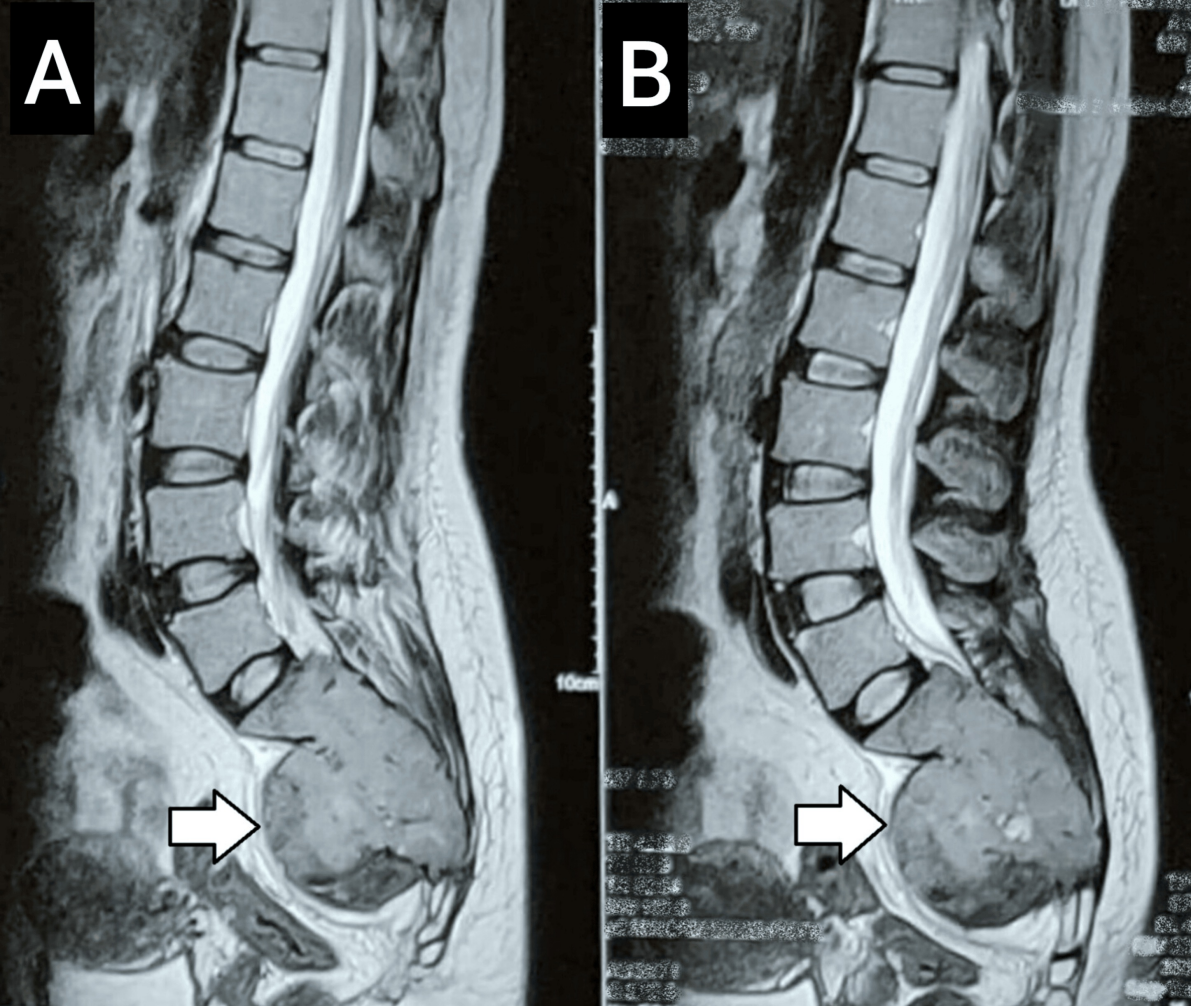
Lumbopelvic MRI in sagittal view demonstrating a lobulated mass lesion (arrow) centered at the sacrum, causing destruction of the sacral bone: (A) non-contrast scan and (B) contrast-enhanced scan
